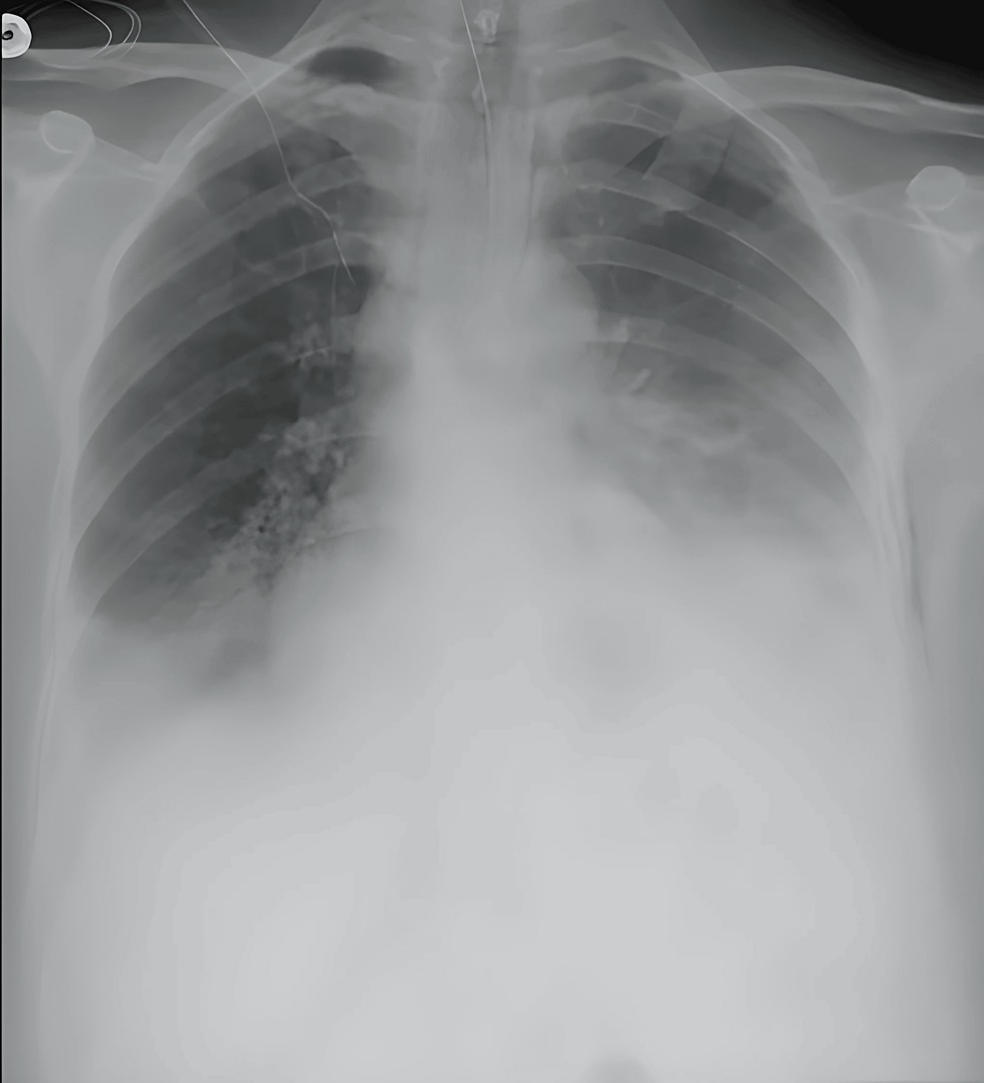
Chest X-ray showing left pleural effusion with pneumomediastinum.
